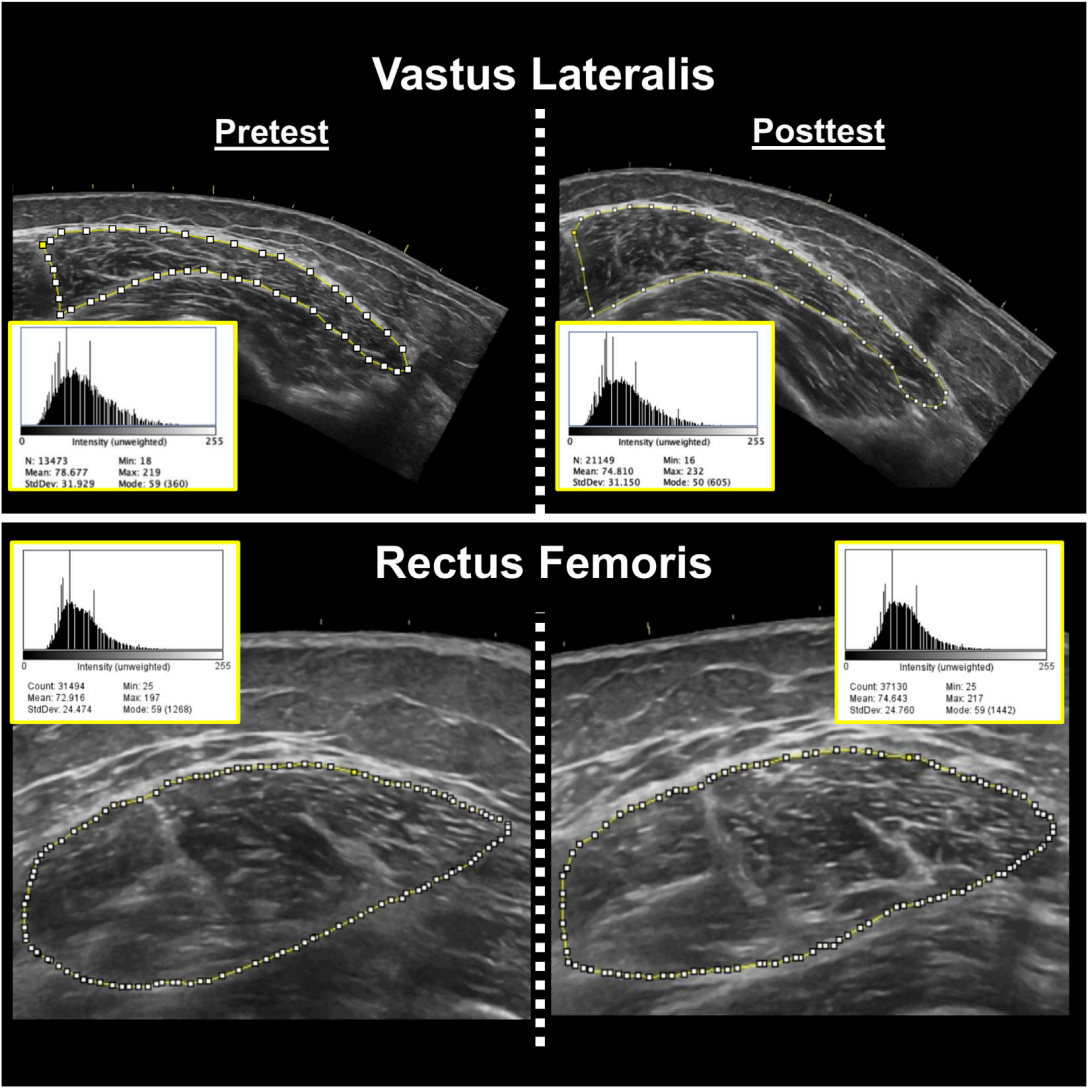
Representative pretest (left) and posttest (right) B-mode ultrasound images of the vastus lateralis (top) and rectus femoris (bottom) muscles from two different participants. Corresponding ImageJ grayscale histograms are shown adjacent to each image, with mean pixel intensity values representing muscle echo intensity. Higher mean values indicate greater intramuscular echogenicity (i.e., reduced muscle quality).These values do not account for subcutaneous adipose tissue thickness. The same investigator conducted all analyses, showed excellent intrarater reliability prior to data collection, and was blinded during analysis.
